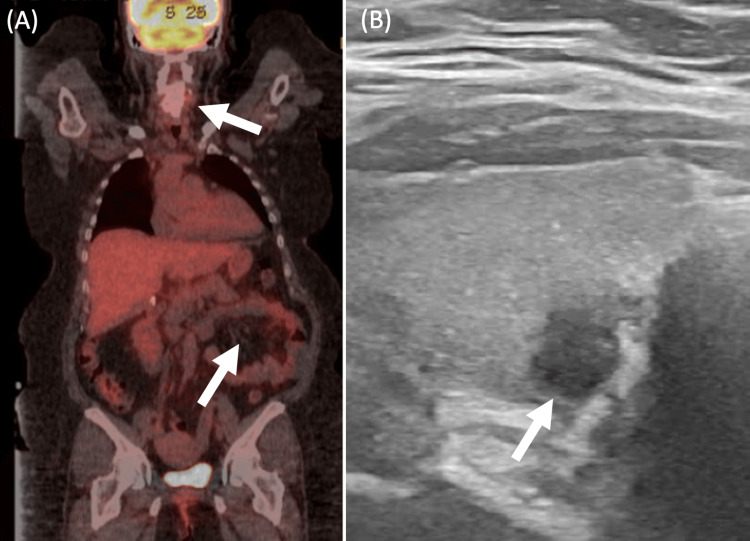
Imaging work-up for suspected gastric cancer demonstrates diffuse gastric uptake on positron emission tomography (PET) CT and a thyroid nodule on PET CT and ultrasound (US). Panel A shows the patient's full body PET CT scan with non-specific diffuse uptake in the stomach and right lobe of the thyroid. Panel B is a follow-up thyroid US revealing a hypoechoic solid nodule with indistinct margins and a widened shape measuring 0.7x0.7x0.6 cm in the posterior interpolar right thyroid.
